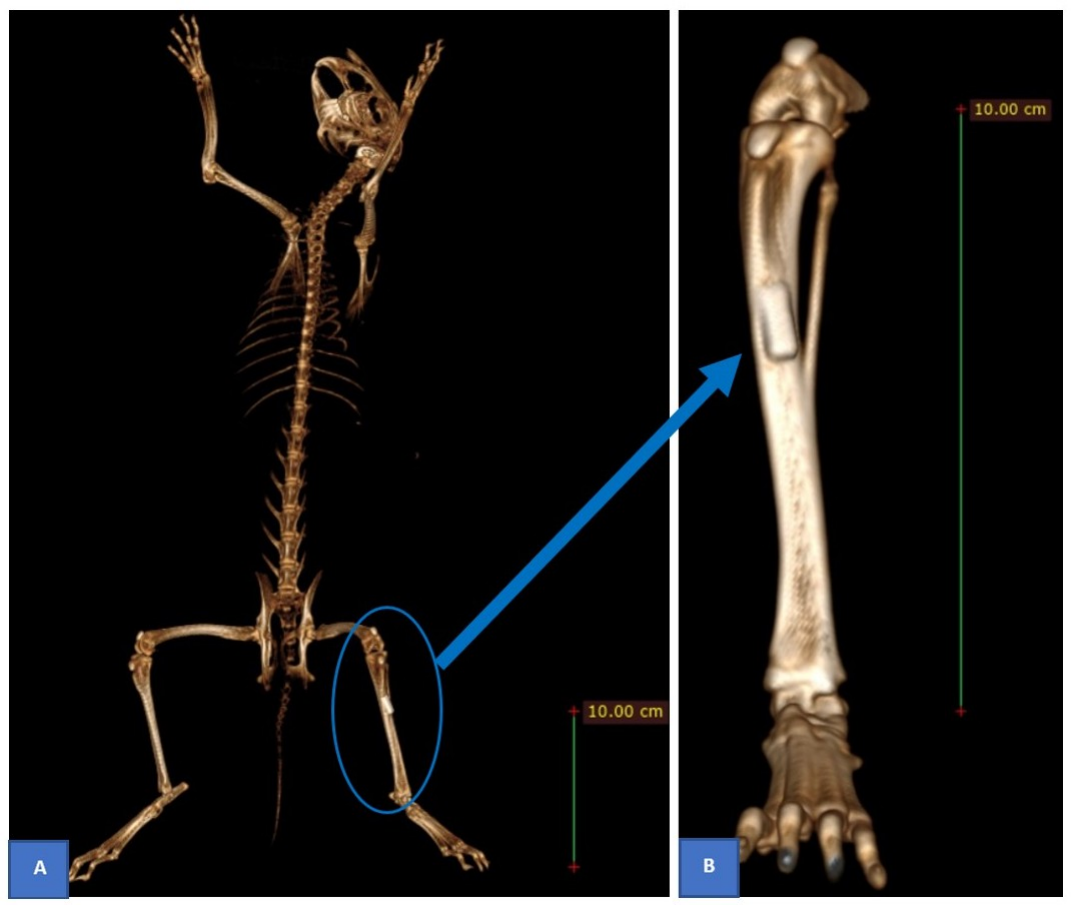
Postoperative control CT-scan reconstruction was obtained to confirm the position of the implant (sample 2). Full body (A) and implant position on the left hind leg (B).
